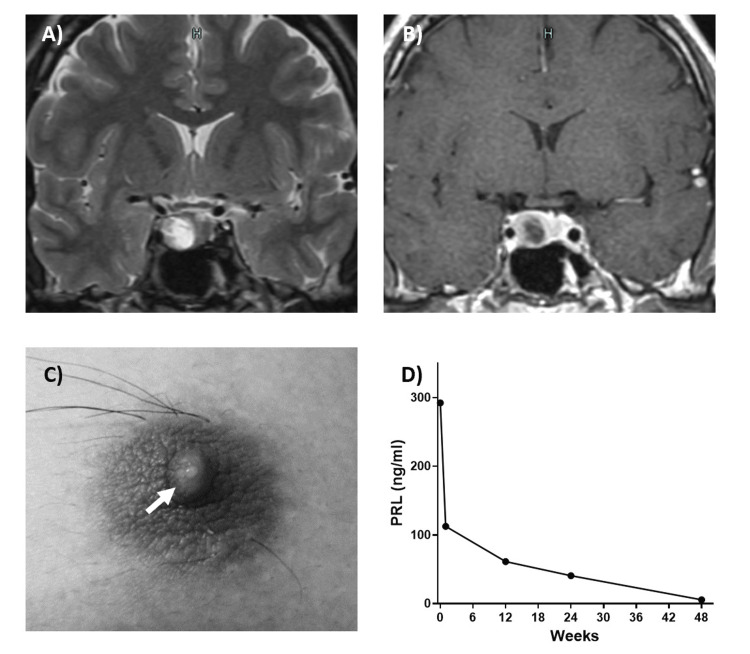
A case of a patient presenting with galactorrhea (Case 7) A: T2-weighted image, coronal section; B: T1 contrast image, coronal section. A neoplastic lesion is seen in the sella turcica; C: galactorrhea (arrow); D: Changes in PRL values. Cabergoline is causing a rapid improvement. PRL: prolactin
